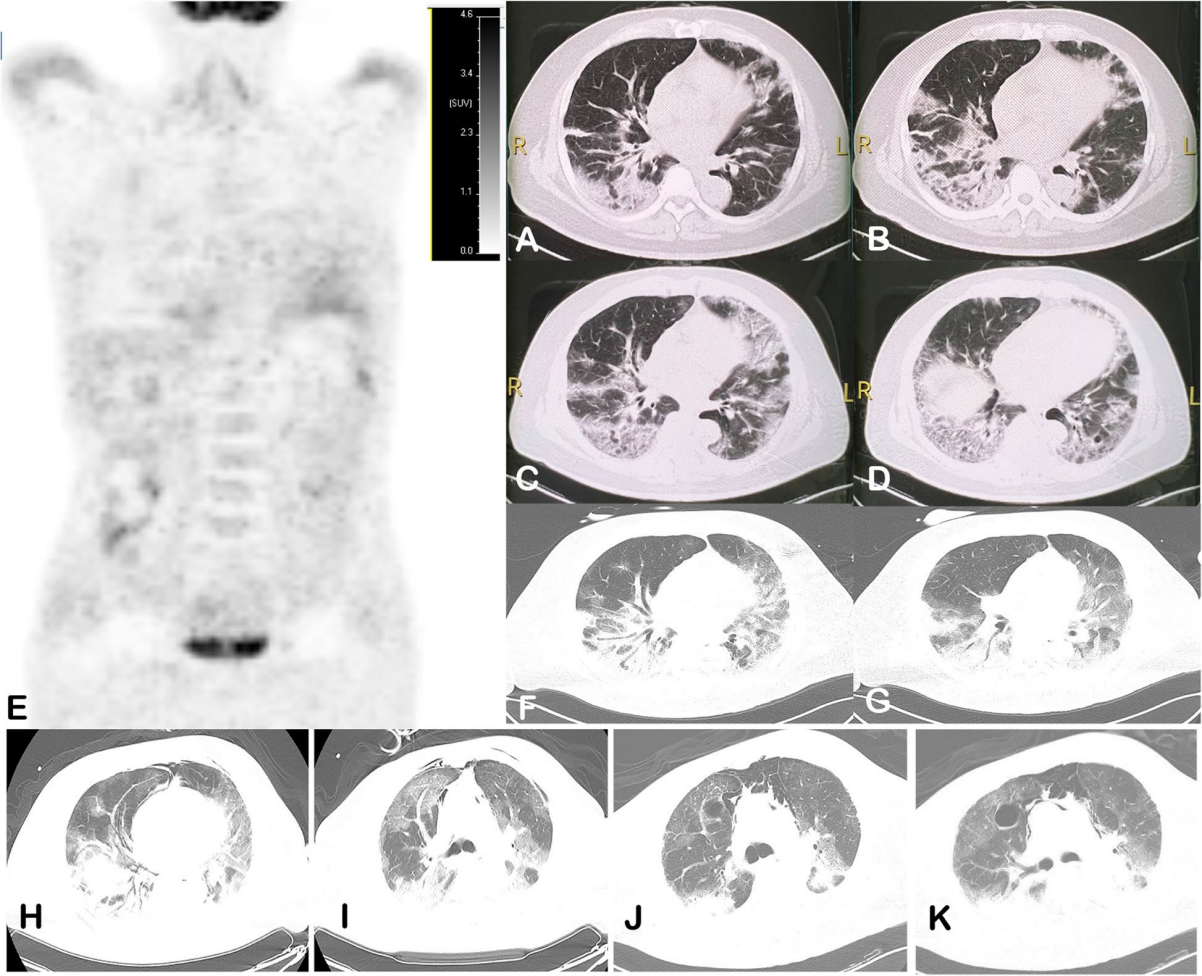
HRCT of the chest and whole body PET-CT fluorodeoxyglucose uptake Imaging. A, B: First visit to the previous hospital, CT showed ground glass opacities in both lobes of the lung. C, D: 1 week before treatment with cotrimoxazole, CT showed increased inflammatory exudate in both lungs with lattice-like and pavement-like lesions. E: Benignly increased FDG uptake in multiple muscles, no abnormal FDG uptake in tumour. F, G: Respiratory distress worse referral to ICU, CT showed inflammation in both lungs with some solidity and bronchial inflation signs. H, I: Complicated bacterial infections after intensive immunosuppressive therapy, CT showed no significant improvement in inflammation in both lungs, solid lung changes and significant interstitial inflammation. J, K: After upgrading antibiotic therapy, before evacuating ECMO, pathological finding was CMVP
